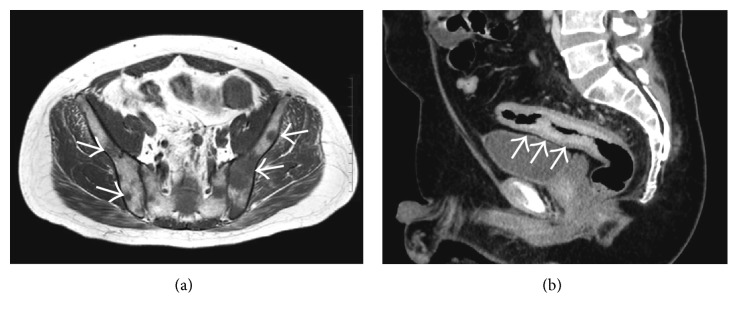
(a) T1-weighted MRI of the pelvis demonstrates multiple lesions in the ilium with low T1 signal intensity (arrows), suggesting multiple bone metastases. (b) Abdominal contrast-enhanced CT scan reveals long segmental bowel thickening in the rectum (arrows).
